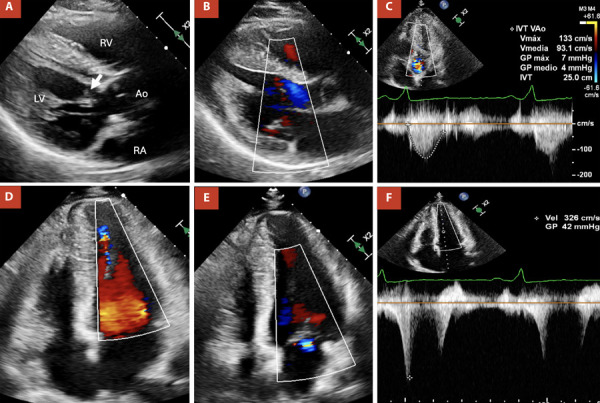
A) Parasternal long-axis view showing redundant chordae tendineae (arrow). B) Parasternal long-axis view with color Doppler, demonstrating the absence of subaortic obstruction (Laminar flow). C) Apical five-chamber view with color and continuous-wave Doppler, showing no evidence of subaortic obstruction. D) Four-chamber view with color Doppler, revealing turbulence in the apical portion of the left ventricle. E) Four-chamber view showing mild mitral regurgitation. F) Four-chamber view with color and continuous-wave Doppler demonstrating apical obstruction with a maximum gradient of 42 mmHg, partially contaminated by mitral regurgitation flow.
